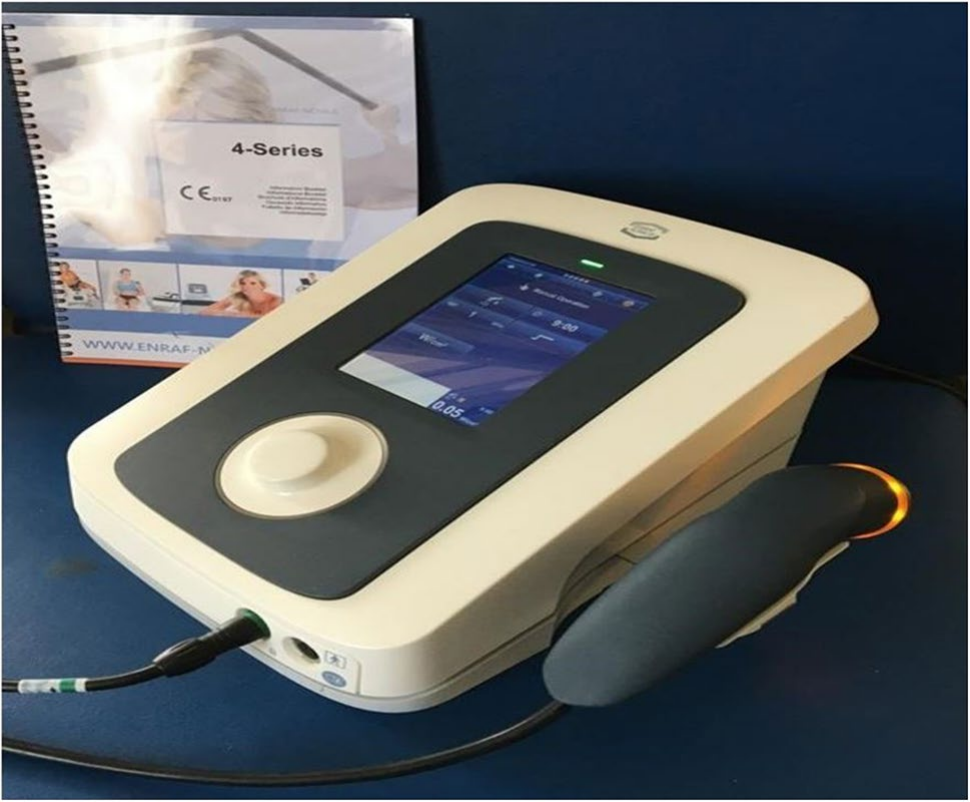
Sonopuls 490 ultrasound therapy device
